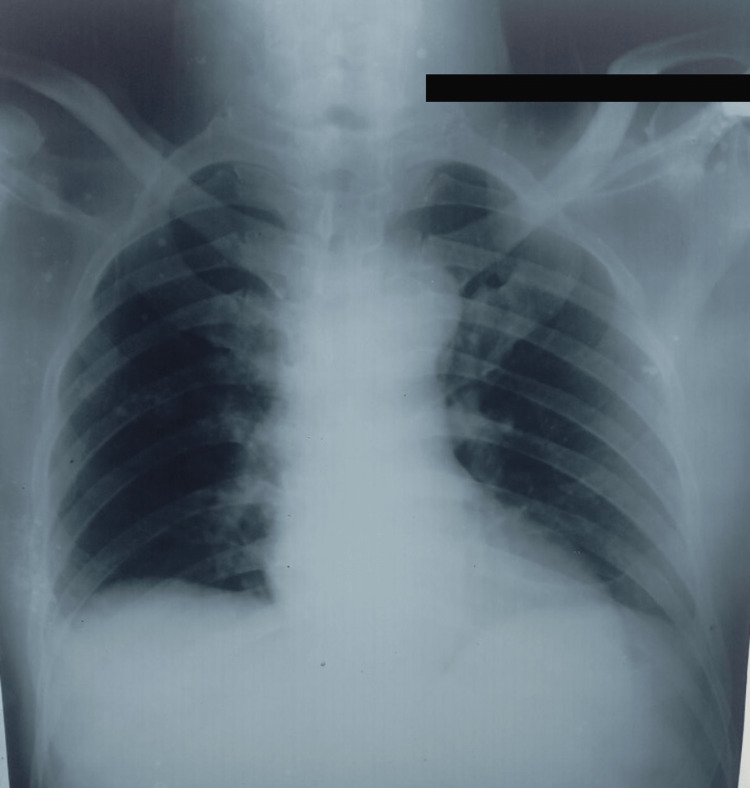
X-ray image of the patient
